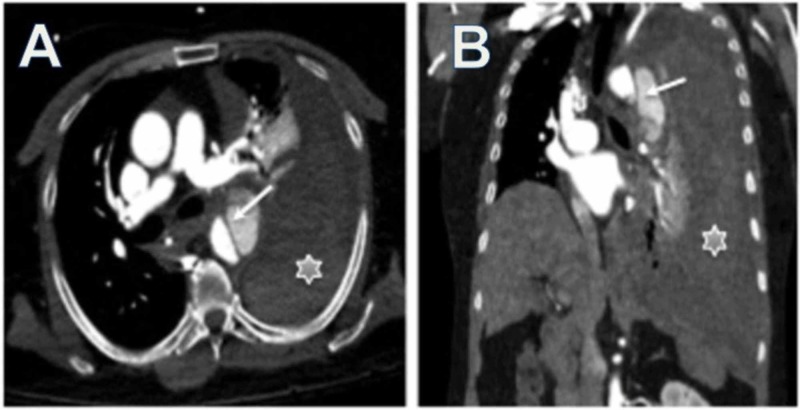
Type B Aortic Dissection Axial oblique (A) and coronal oblique (B) reformats and 3D volume rendering image of thoracic CT angiography images demonstrate Stanford type B thoracic aortic dissection (arrow) and rupture, with left hemothorax, pleural effusion, and near complete atelectasis of the left lung (star).
